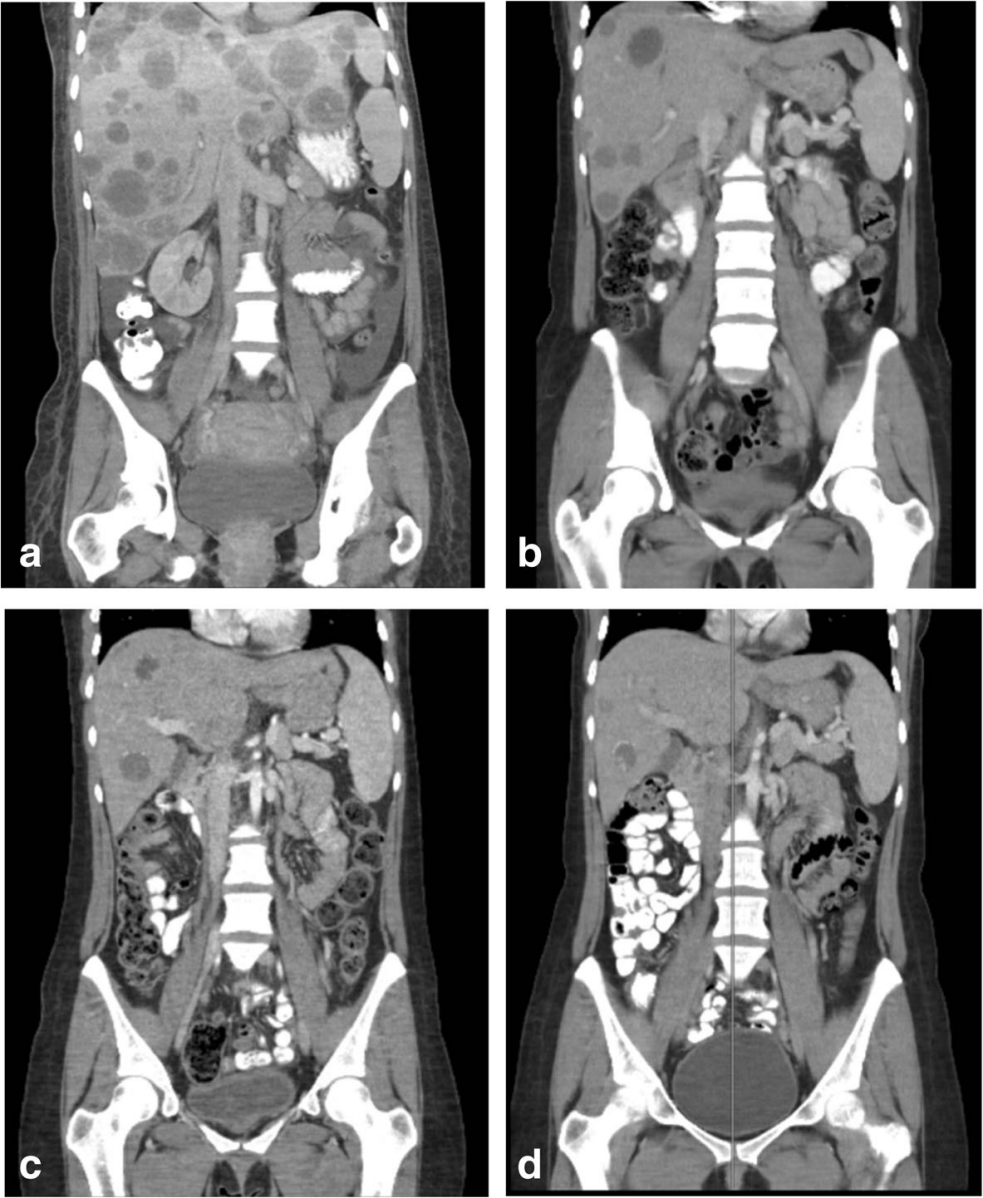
Radiographic evaluation of metastatic disease. a Baseline prior to initiating chemotherapy. b After five cycles of etoposide + methotrexate + actinomycin D ± cyclophosphamide + vincristine (EMA/CO) therapy. c After eight cycles of EMA/CO therapy. d After 11 cycles of EMA/CO therapy
